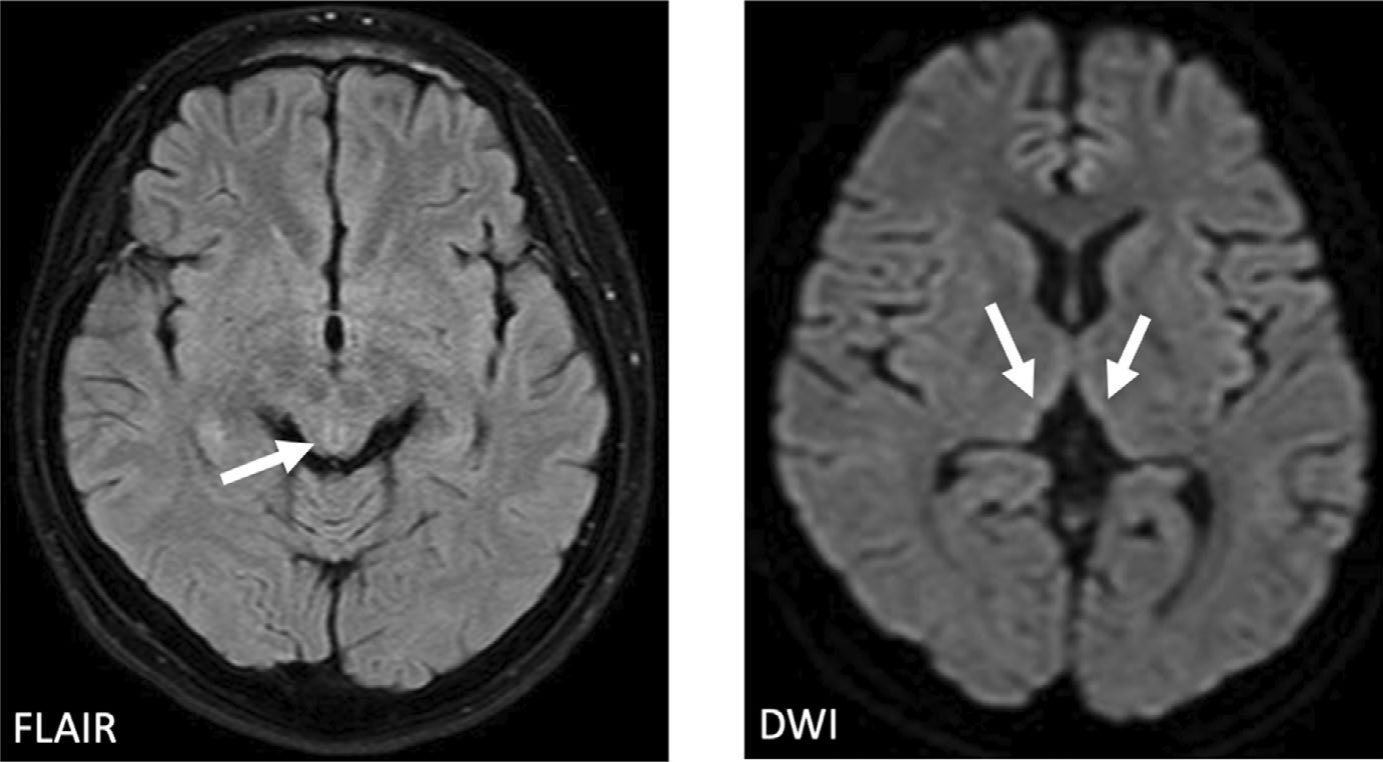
Magnetic resonance imaging (MRI) of the patient's brain. Diffusion‐weighted image (DWI) and fluid attenuated inversion recovery (FLAIR) revealed increased signal intensity in the bilateral dorsomedial thalami and around the cerebral aqueduct (white arrows).
